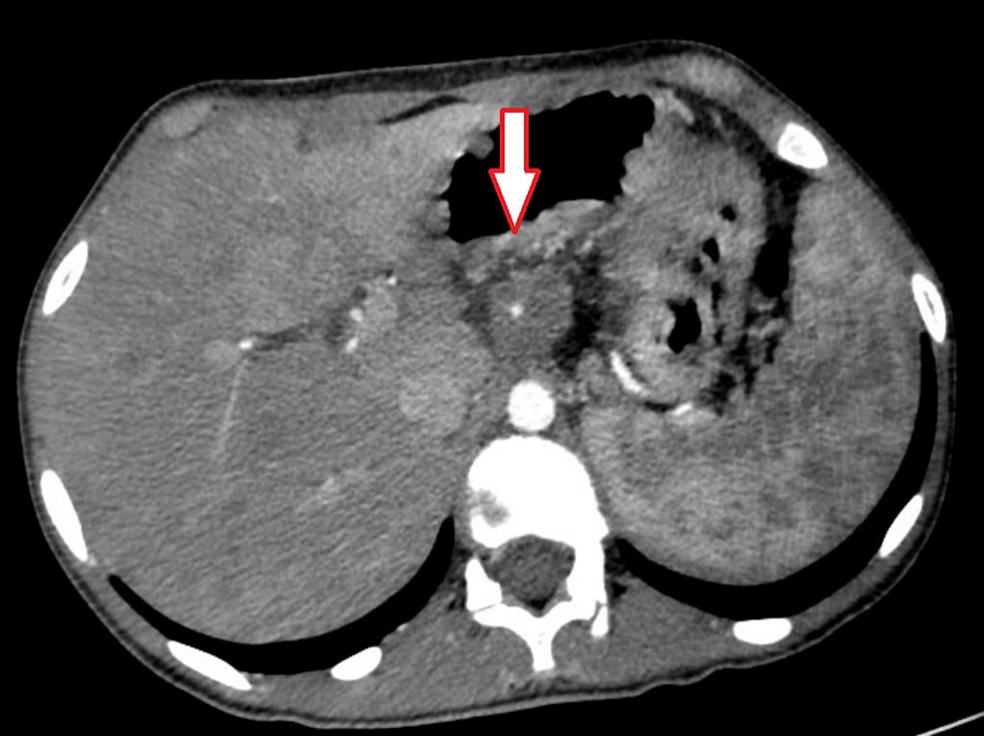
CT abdominal scan revealing GEJ thickening, supporting the presence of a mass GEJ: gastroesophageal junction
